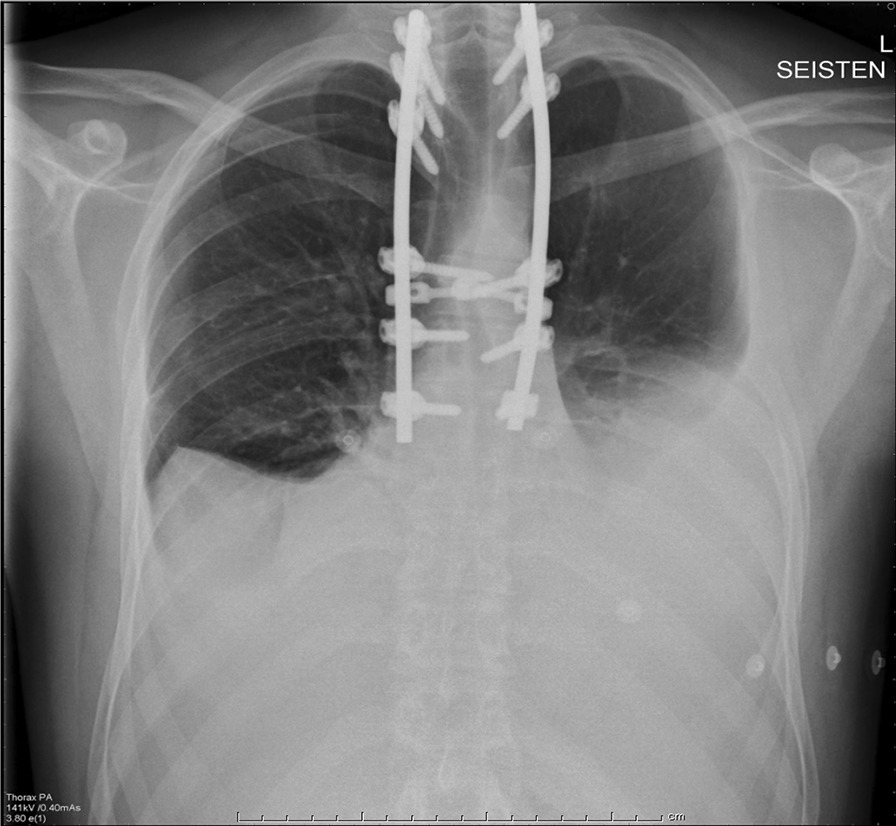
Pleural effusion was revealed on chest x-ray
